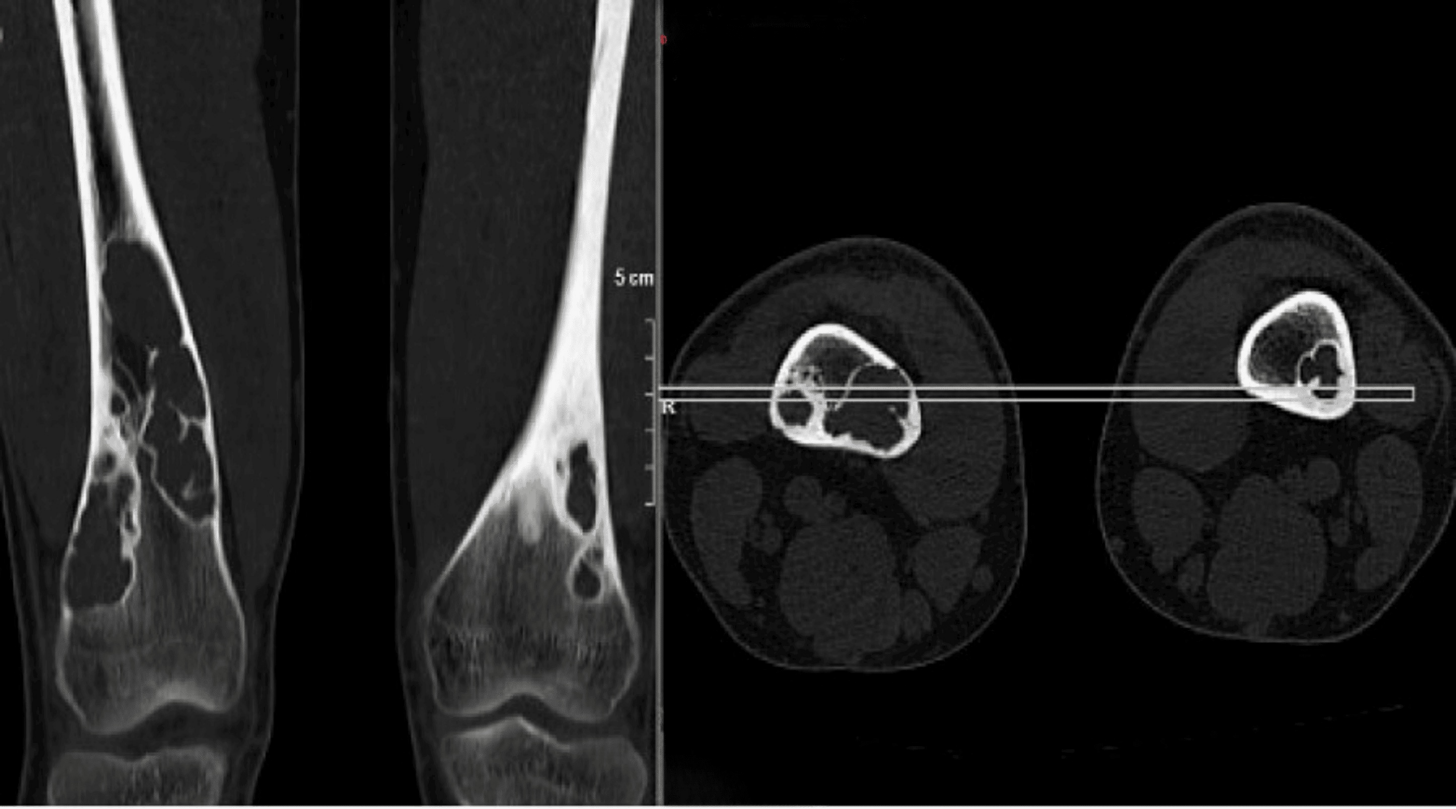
CT images of NOF in both femurs of the patient CT: computed tomography, NOF: non-ossifying fibroma
